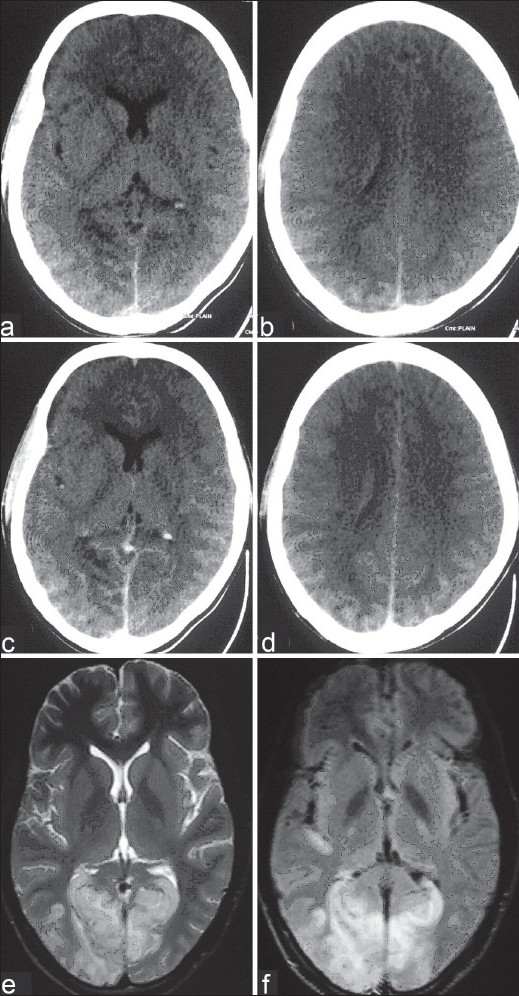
(a–e) : CT scan (2nd December 2007) showing ill-defined bilateral right more than left occipitoparietal interdigitating hypodense lesions with effacement of adjacent sulci and no postcontrast enhancement. (e, f) MRI (29th November 2007) shows bilateral right more than left occipitoparietal T2W and FLAIR hyperintense lesions predominantly involving the white matter but also the gray matter in the right occipital area
